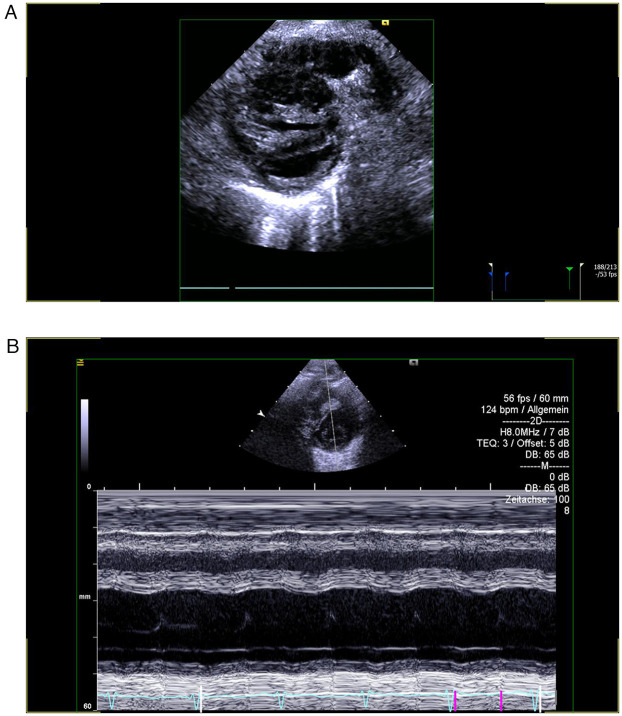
(A, B) Transthoracic echocardiography, two-dimensional and M-mode, of normal right ventricular contractility, flattening of the interventricular septum, and virtual akinesia of the left ventricular free wall.
